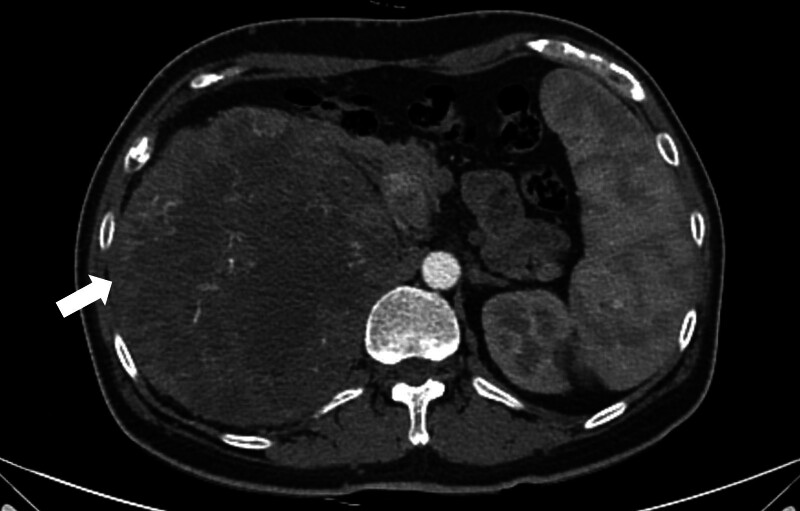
Abdominal computed tomography, axial view, of this patient, showing a heterogeneous enhancing mass (arrow) replacing the right kidney with nephromegaly (long diameter, 19.1 cm), with the mass extending into the renal pelvis and proximal ureter, suggesting a malignant renal tumor.
